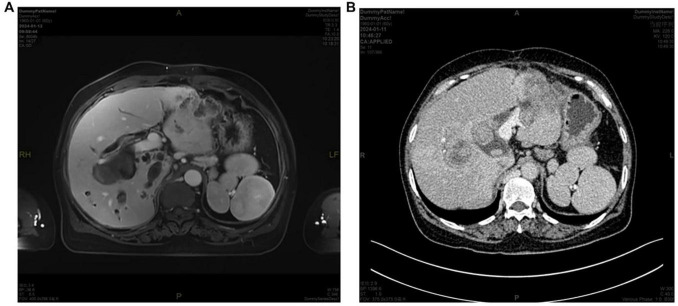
(A) MRI and (B) CT images demonstrating multiple spleens.
